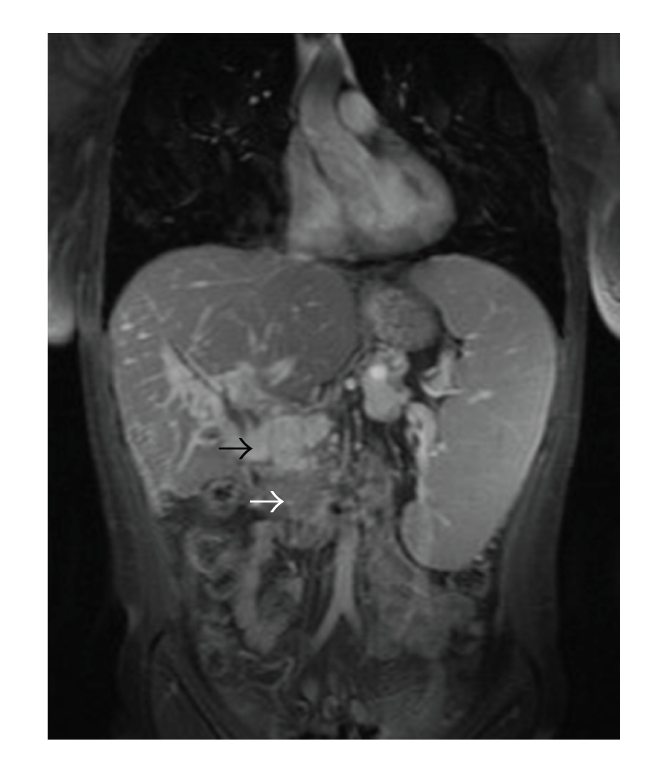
Coronal MRI T_2_ weighted image showing a solid tumor-like mass at the hepatic hilum (portal cavernoma), (white arrow) in addition to hyposignal (thrombus) at the level of mesentiricoportal confluence (black arrow).
